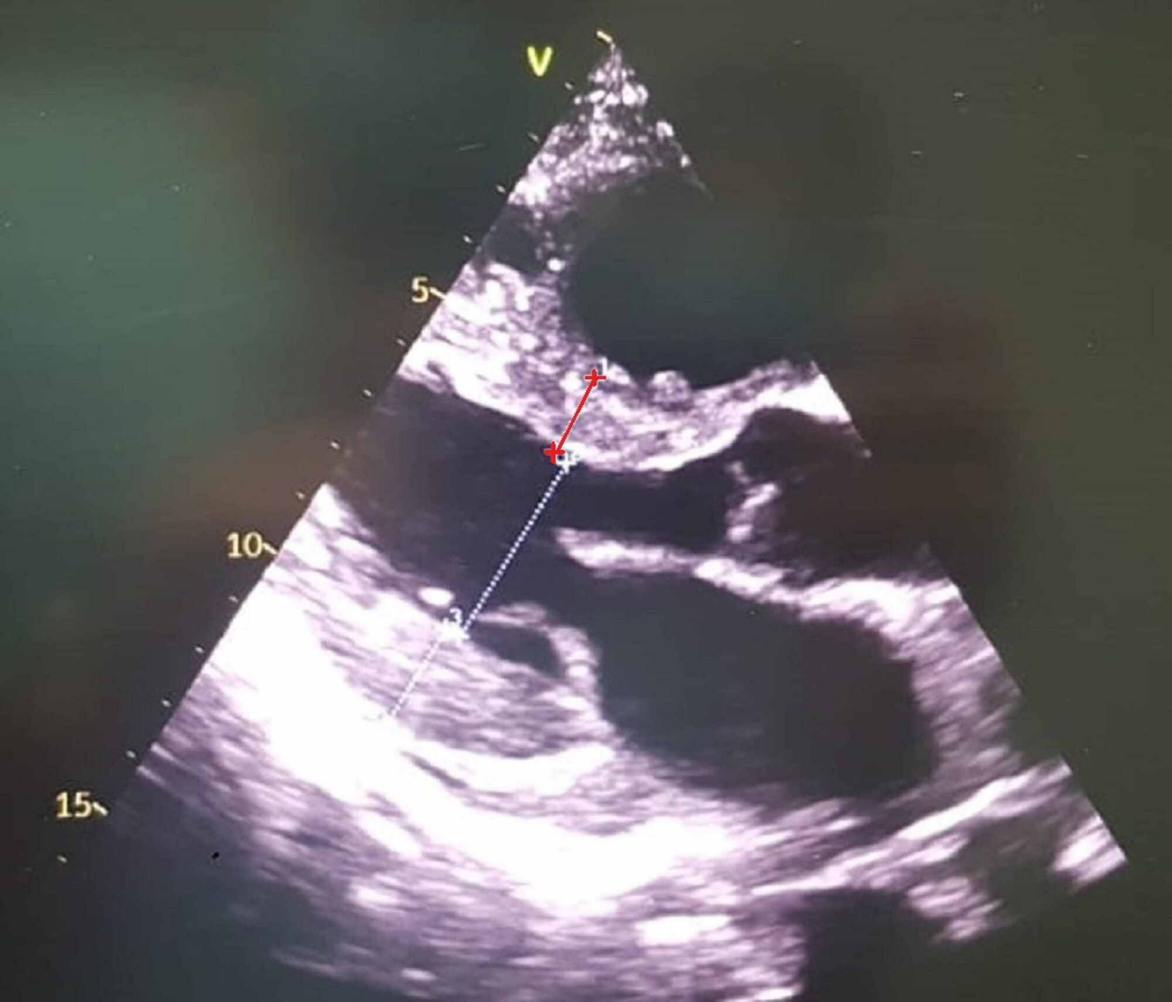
Parasternal long axis view echocardiography showing septal hypertrophy Red line: Septal hypertrophy
